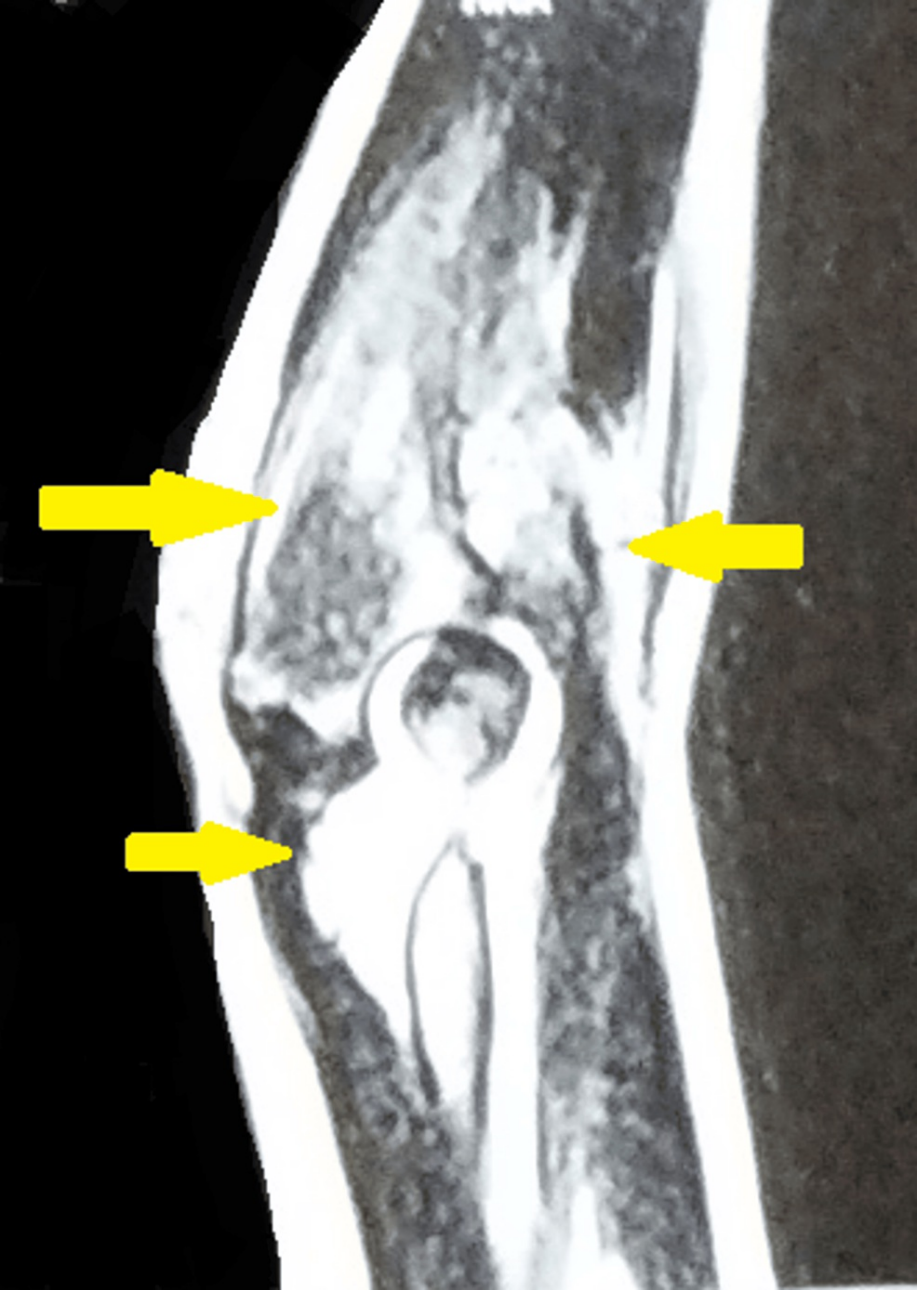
MRI of the right elbow was suggestive of altered signal intensity in the medial and lateral humeral condyle and epicondyle, the olecranon process of the ulna, trochlea, and radial head, and the opposing surfaces of the proximal radioulnar joint with partial loss of definition and widening of elbow joint space MRI: Magnetic resonance imaging
